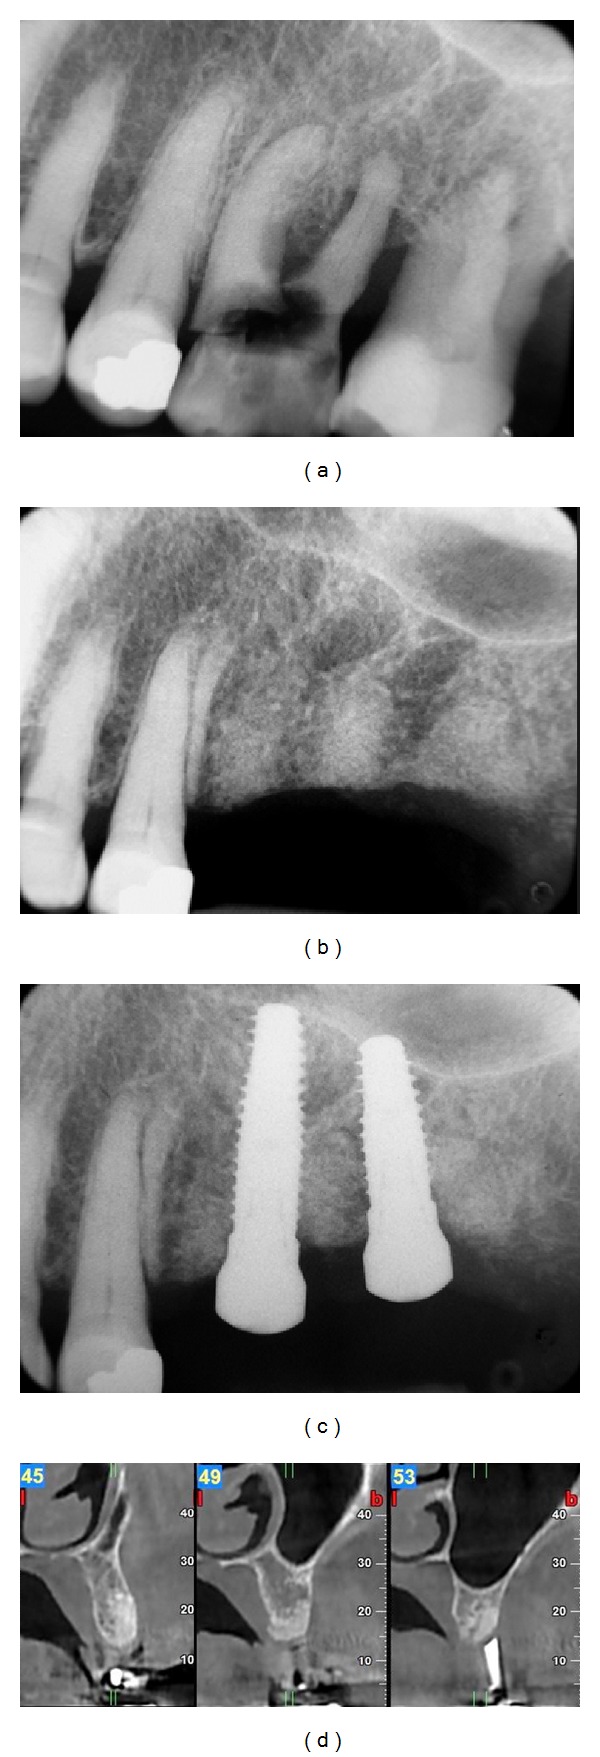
Radiographic views of Case  1. Periapical radiographs (a) before extractions, (b) at 6 months, and (c) after implant placement. (d) CBCT images at 6 months postoperatively.
